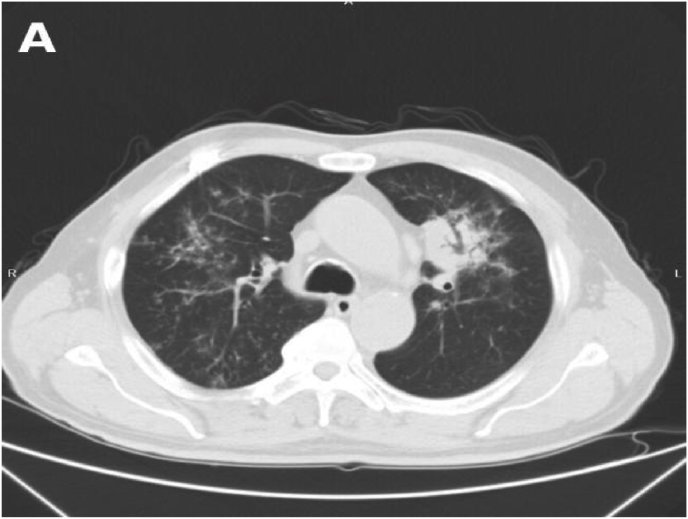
Follow-up chest CT on Day 26 (D26). (A) Chest CT obtained on Day 26 (D26) showing progression of the lesion in the left upper lobe, with partial resolution of infiltrates in both lower lobes compared with initial imaging.
